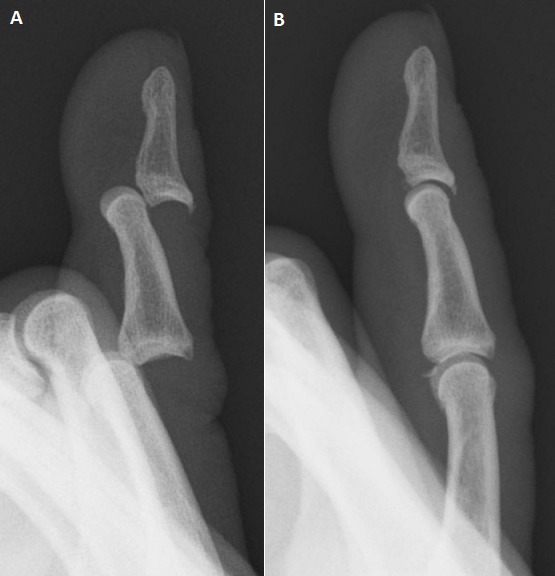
Case 2 (A) X-rays of the little finger showing dorsal dislocation of both interphalangeal joints; (B) Post-reduction X-rays with a volar rim fracture
